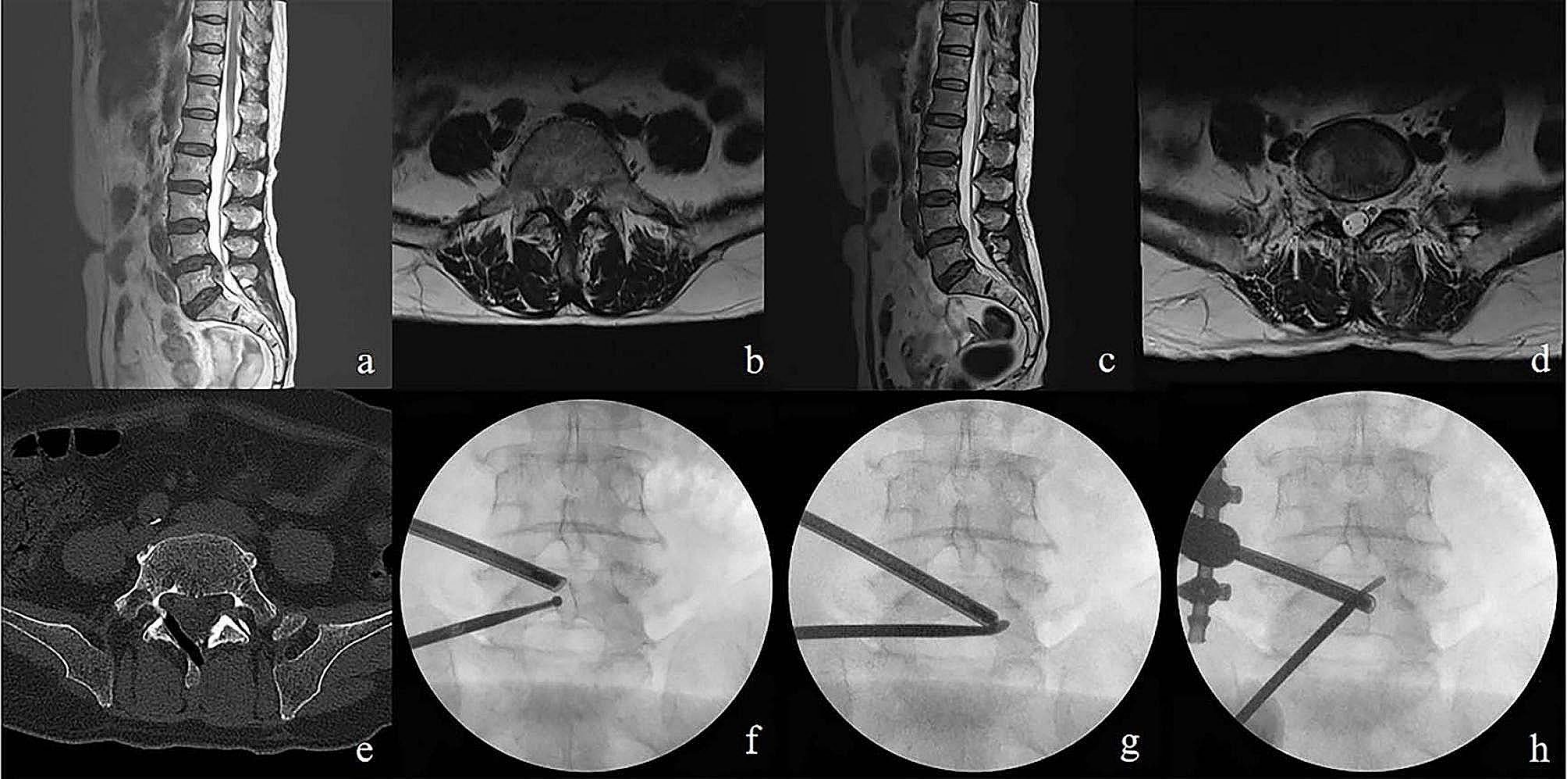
L4/5 down migrated disc shown in preoperative T2-weighted sagittal magnetic resonance imaging in sagittal (a) and axial views (b). Complete removal of ruptured disc without remnant disc material in postoperative MR images in sagittal (c) and axial views (d). Keyhole in contralateral spinolaminar junction without violation of facet joint shown in postoperative CT image (e). Desired initial tagert portal position in X-rays (f). Intraoperative fluoroscopicimage confirming inferior (g) and superior (h) of pedicle and complete decompression of traversing nerve root
